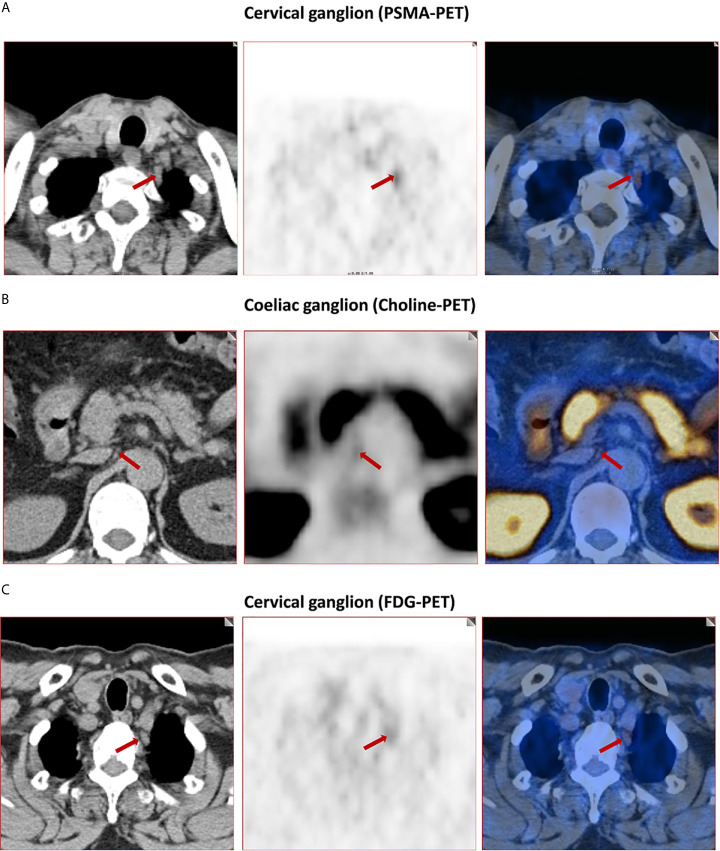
Representative images of PSMA-positive, choline-positive and FDG-positive ganglia. (A) PSMA-positive cervical ganglia (red arrow, 4.8 SUVmax for PSMA-PET). (B) Choline-positive coeliac ganglia (red arrow, 2.8 SUVmax for choline-PET). (C) FDG-positive cervical ganglia (red arrow, 2.9 SUVmax for FDG-PET).
